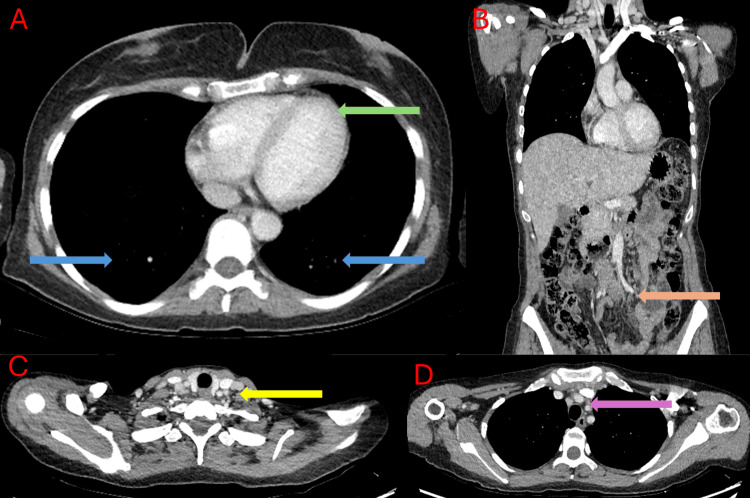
One-year follow-up contrast-enhanced CT showing complete radiological remission. (A) Axial chest CECT image showing resolution of pleural effusion (blue arrows) and pericardial effusion (green arrow). (B) Coronal abdominal CECT image demonstrating absence of ascites (orange arrow). (C) No evidence of cervical lymphadenopathy (yellow arrow). (D) Complete regression of the previously noted prevascular mediastinal mass (red arrow).
